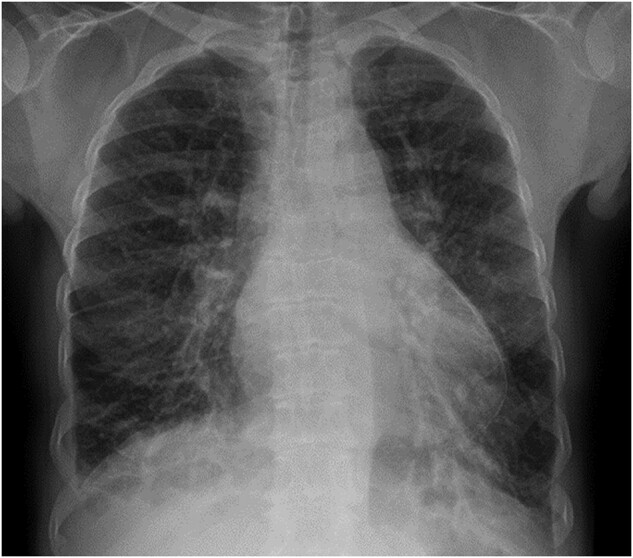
Initial chest x-ray demonstrating pericardial calcification.
